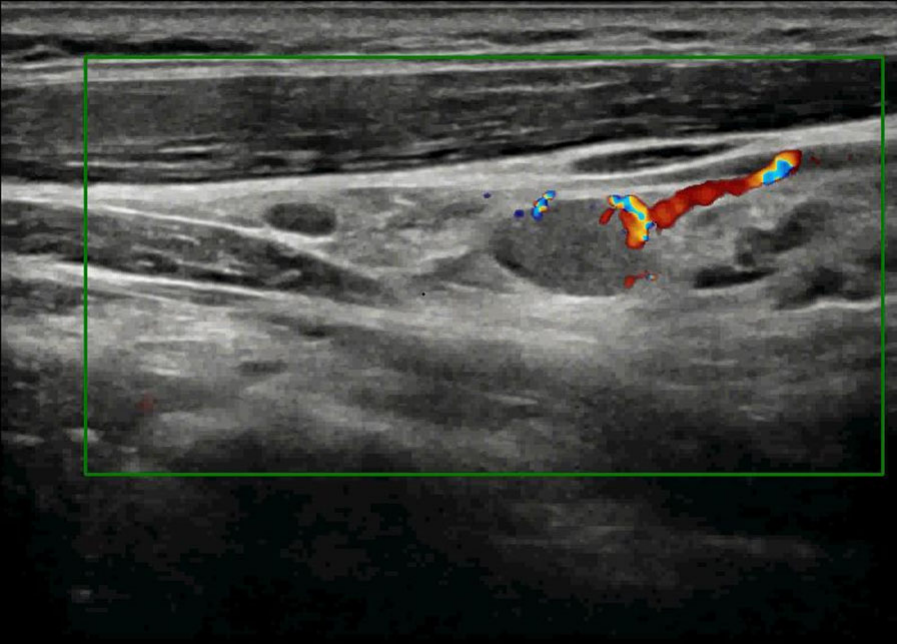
Color Doppler ultrasound demonstrates metastatic involvement in cervical region III, revealing sparse peripheral blood flow signals within the lesion.
